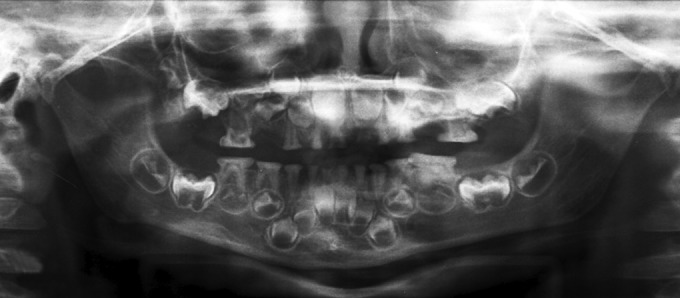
Orthopantomogram X-ray with severely attrited deciduous teeth with obliterated pulp chambers
